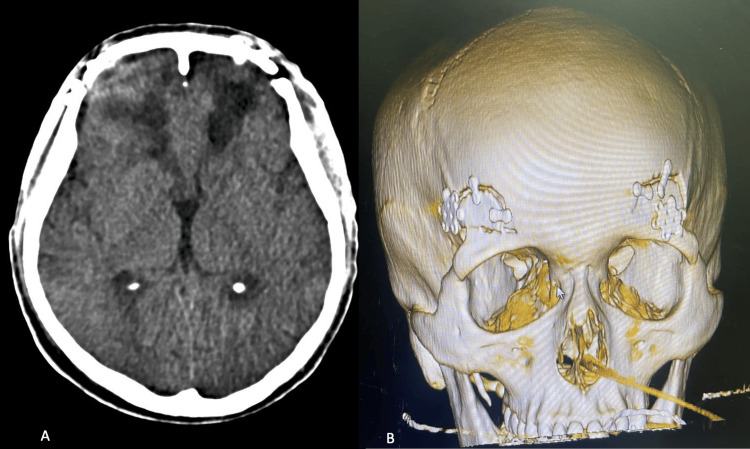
(A) Follow-up CT scan after evacuation of the hematoma, (B) 3D bone reconstruction of the bilateral supraorbital craniotomy
